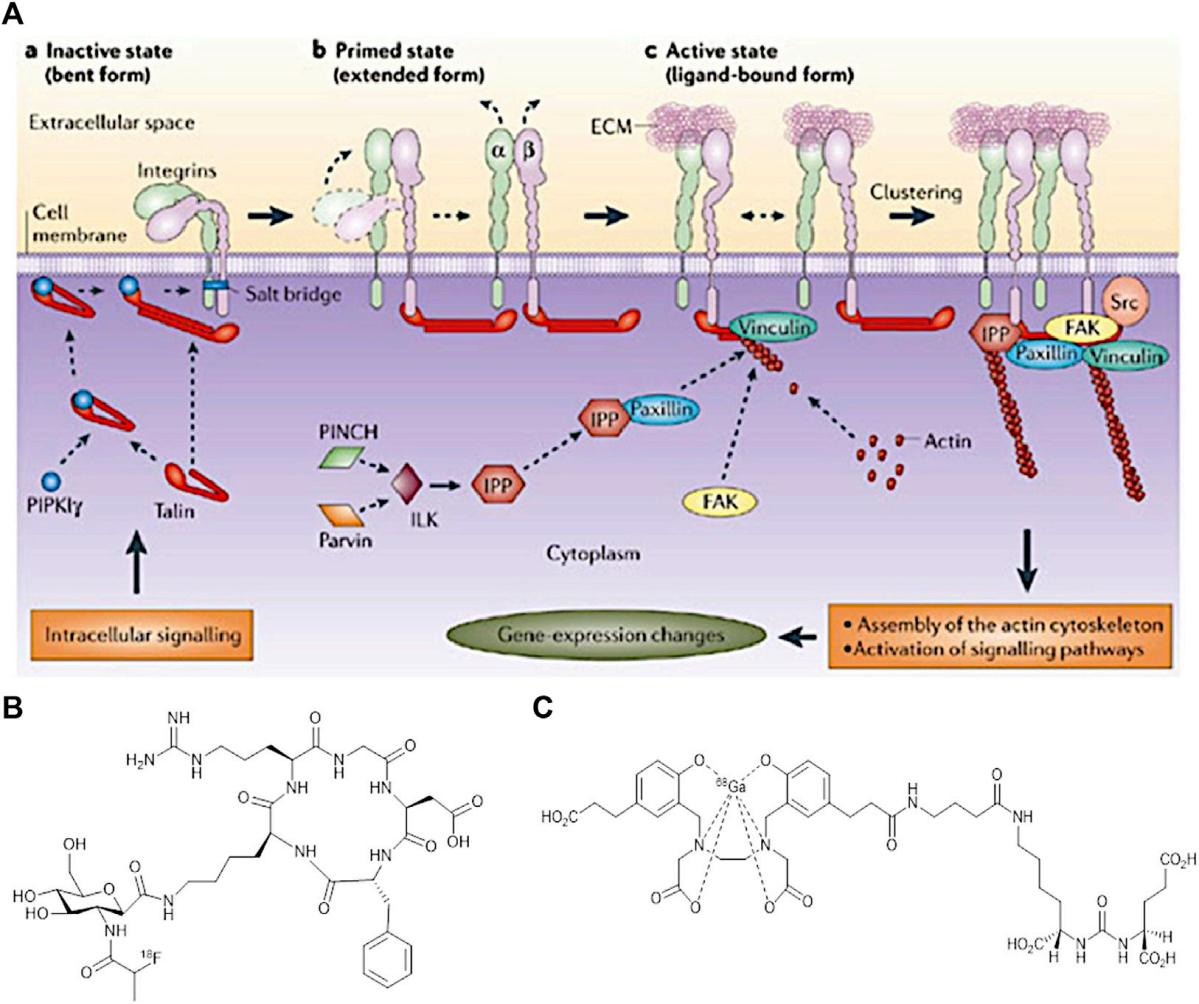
(A) Interaction of integrins with extracellular and intracellular molecules (Backer and Backer, 2012) (B) Structure of [^18^F]Fgalacto-RGD PET Radiotracer, and (C) The structure of [^68^Ga]GaPSMA-11. (Figure adapted from reference (Rudin, 2005)).
